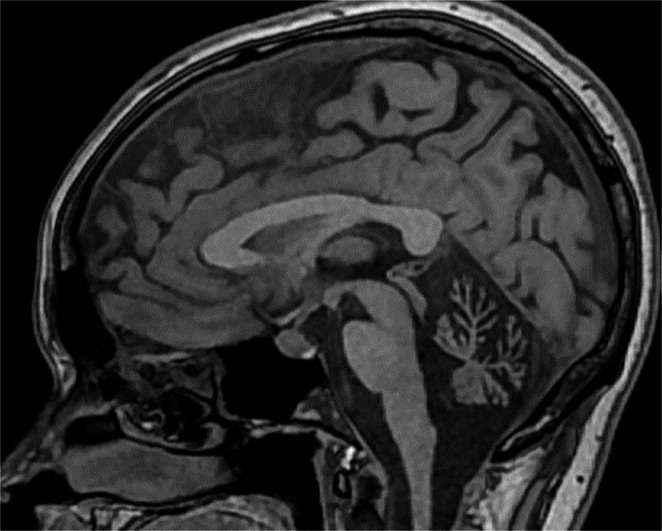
Sagittal T1-weighted image showing pronounced cerebellar atrophy, with preservation of supratentorial structures
